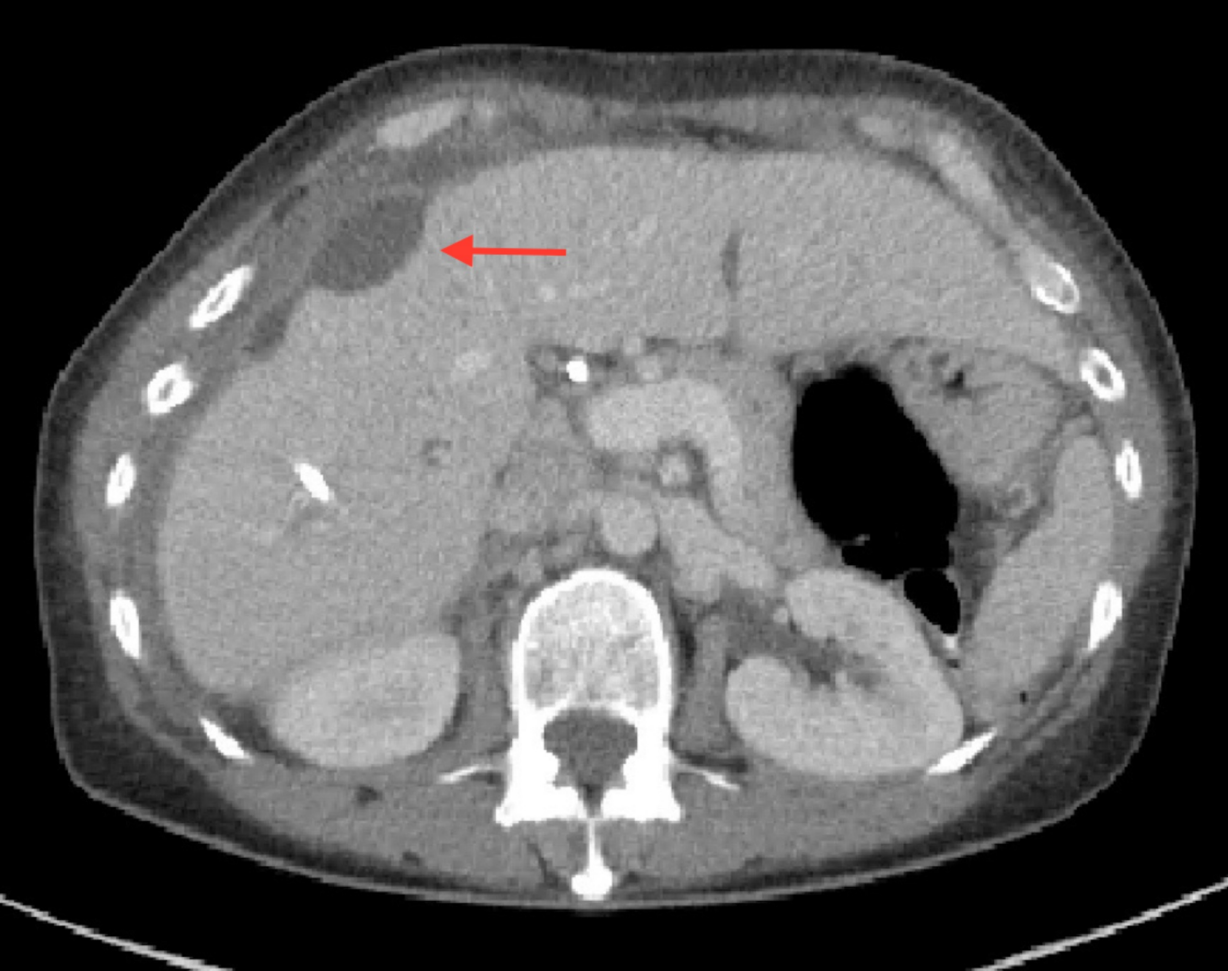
Computed tomography (CT) demonstrating minimal amount of fluid layering adjacent to the liver edge with a small 3-cm focal collection
